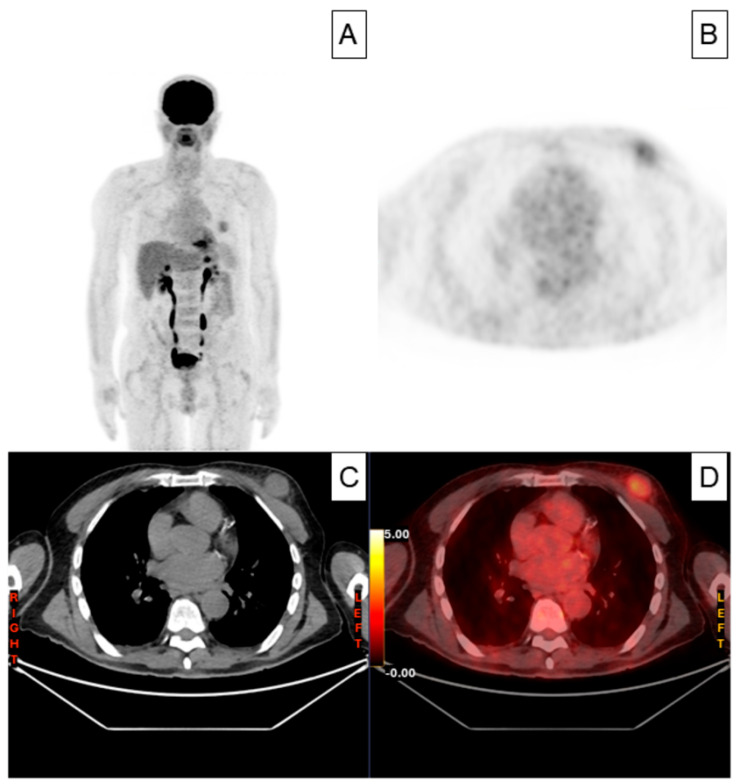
(A–D) 18F-Fluorodeoxyglucose (18F-FDG) positron emission tomography with computed tomography (PET-CT) showing a nodular solid lesion with a faintly increased metabolic standardized uptake value (SUVmax) of 3 located in the upper outer quadrant of his left breast.
